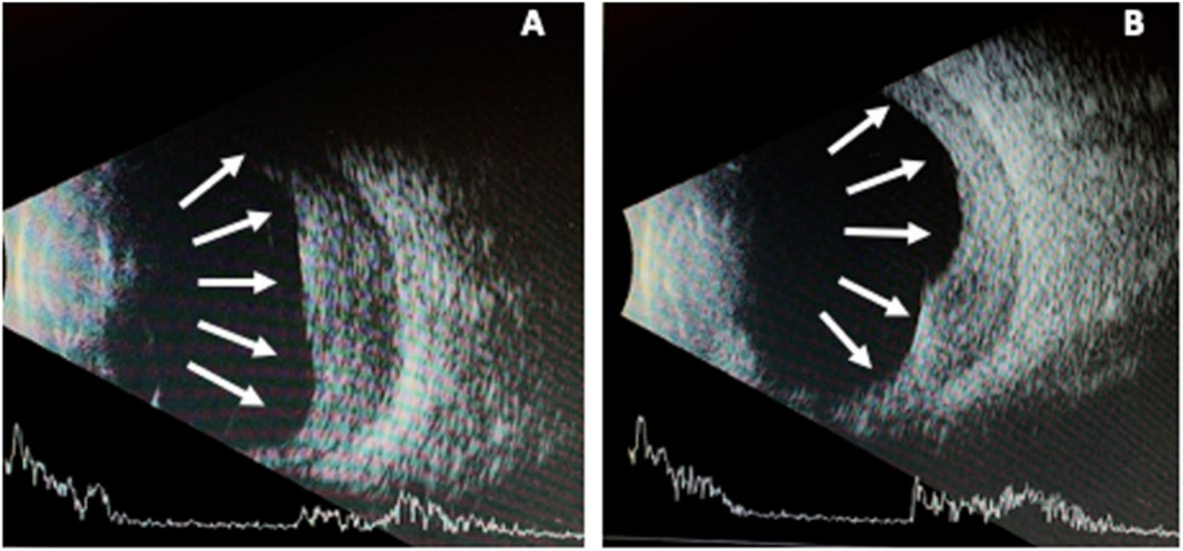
A: B-scan ultrasound of right eye showing diffuse choroidal thickening indicated by arrows. B: B-scan ultrasound of left eye showing diffuse choroidal thickening indicated by arrows
